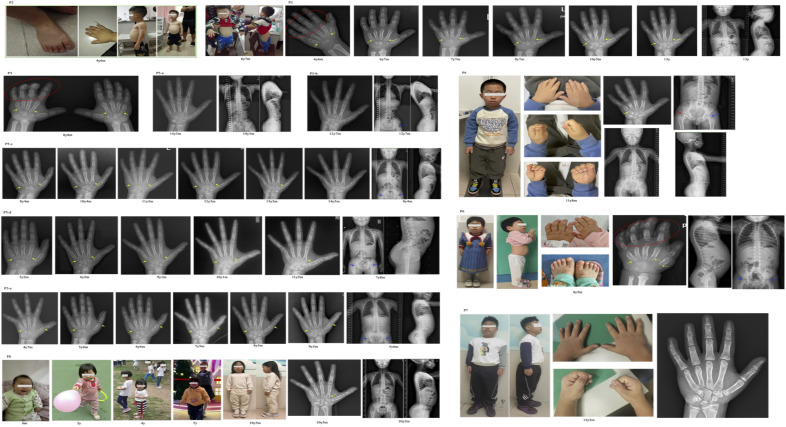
Facial features and radiographic findings of the hand and spine of the AD/GD2 participants. Red elliptical lines indicate conical epiphyses. Red arrows indicate femoral head epiphyseal dysplasia. Yellow arrows indicate the medial notch of the second metacarpal and the lateral notch of the fifth metacarpal. Blue arrows indicate beak-shaped femoral heads.
